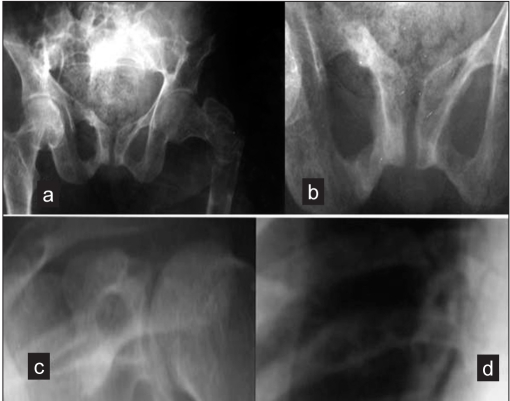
(a) Anteroposterior radiograph of pelvis with both hip joints showing a pathological subtrochanteric fracture with lytic lesion in proximal femur. (b) Loosers zones in pubic rami. (c) Osteolytic lesion in scapula. (d) Osteolytic lesion in ribs
